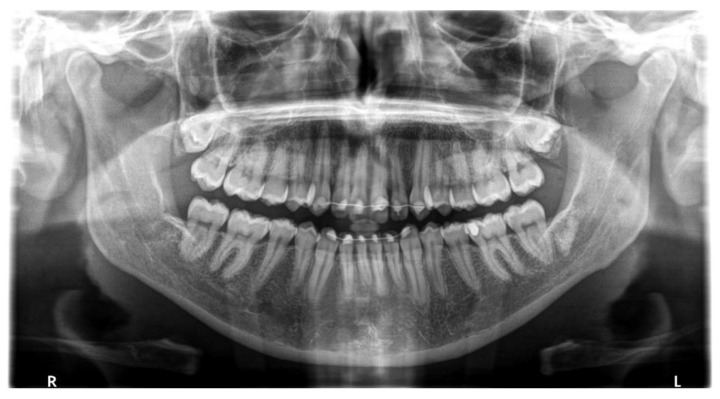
Post-operative panoramic radiographic exam showing bone regeneration in both control and test sites.
